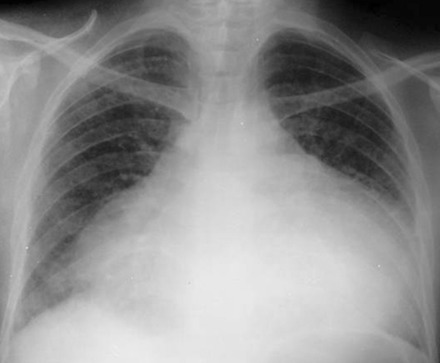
Radiographie du thorax face chez la patiente numéro 1: aspect de cardiomégalie
